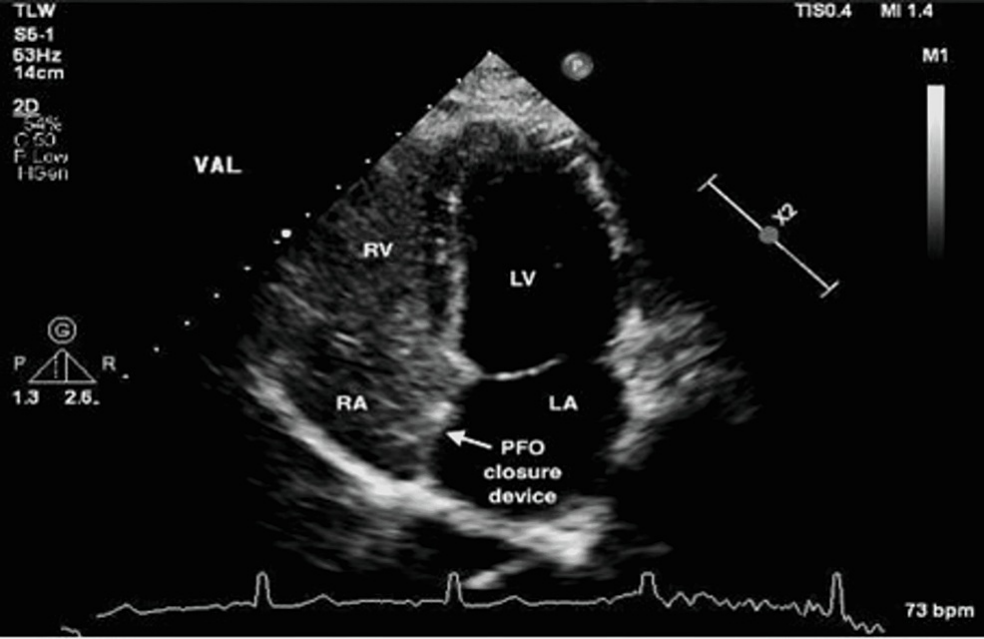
Transthoracic echocardiogram with bubble study post-procedure. Transthoracic echocardiogram and bubble study conducted after the closure procedure showing a negative bubble study and the occluder device in the correct location. Beats per minute (BPM), Left atrium (LA), Left ventricle (LV), Patent foramen ovale (PFO), Right atrium (RA), Right ventricle (RV), Valsalva (VAL).
